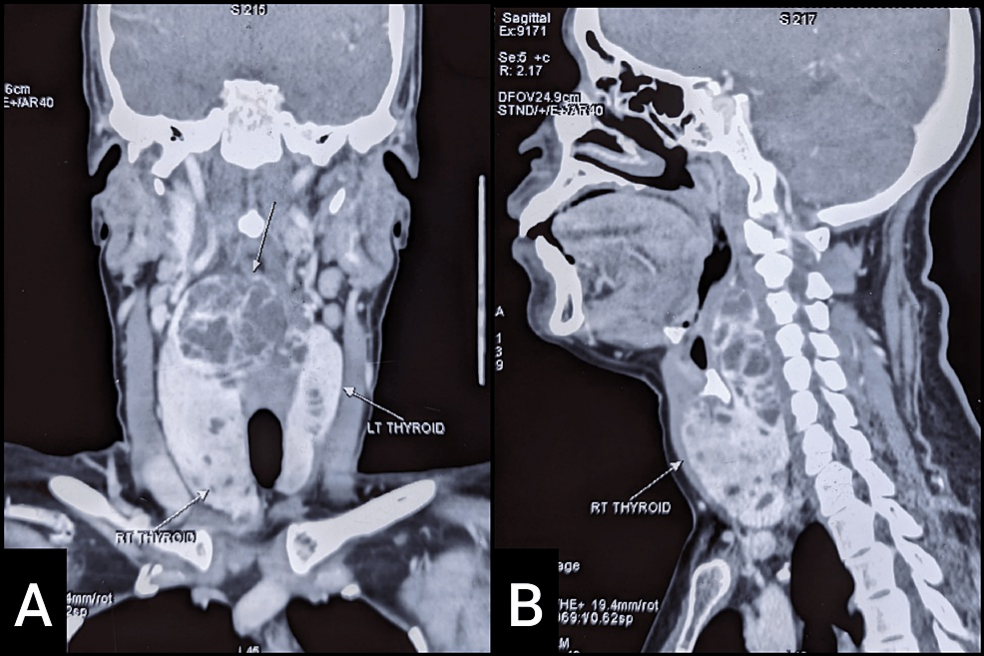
The contrast-enhanced computed tomography (CECT) of neck. (A) Coronal plane showing diffusely enlarged thyroid gland with the right lobe larger than the left lobe. (B) Sagittal plane showing enlarged right lobe of thyroid gland.
